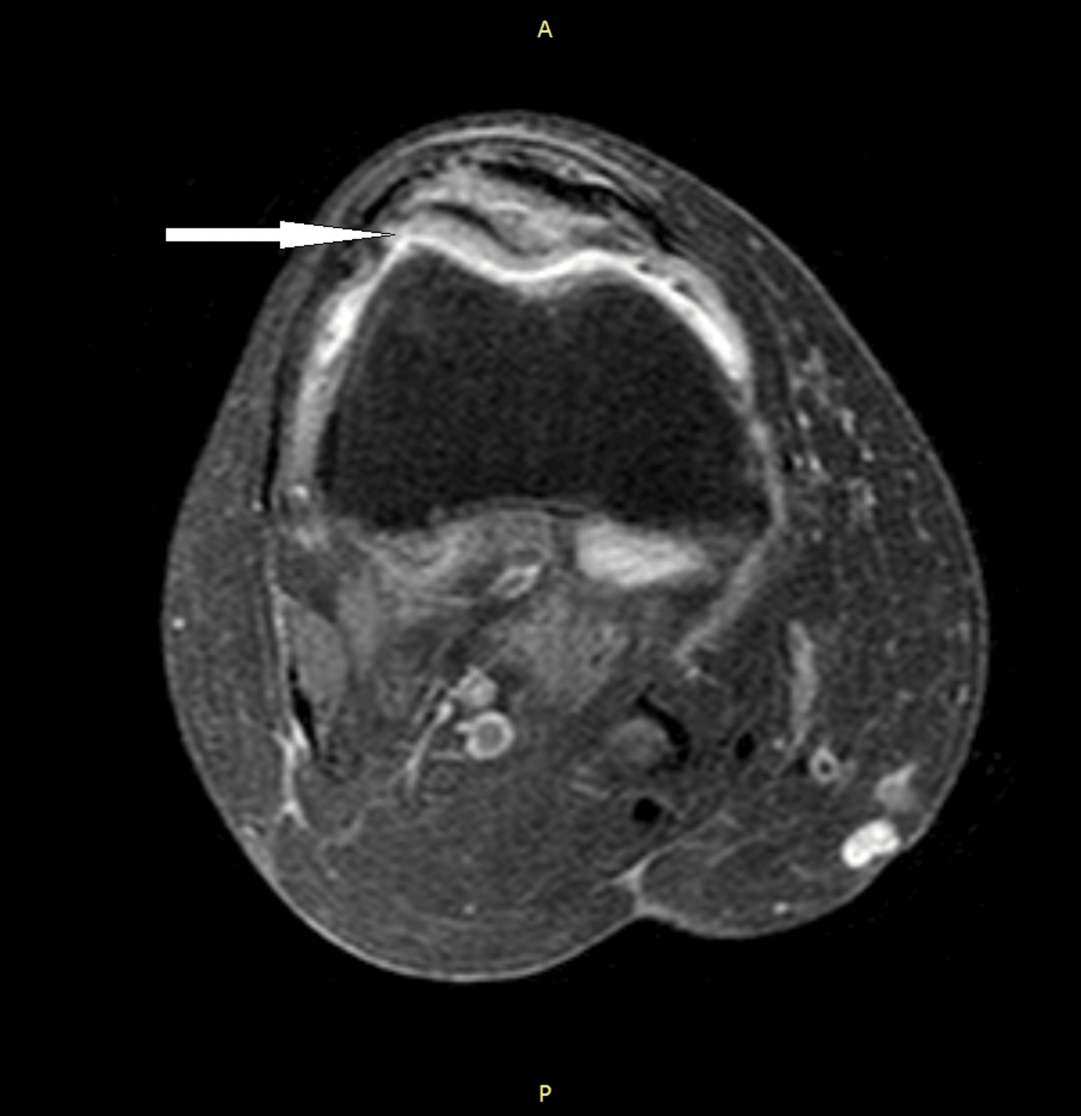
Axial view T2-weighted proton density inversion recovery MRI showing an increased signal seen within the medullary cavity of the patella demonstrating inflammation.
